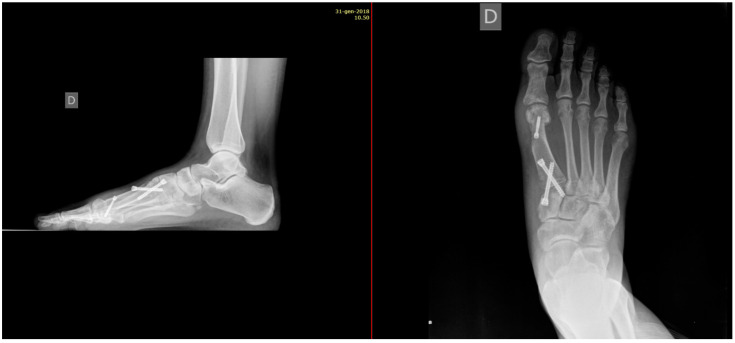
Post-operative weight-bearing X-rays of the foot: lateral view (left) and dorsoplantar view (right).
